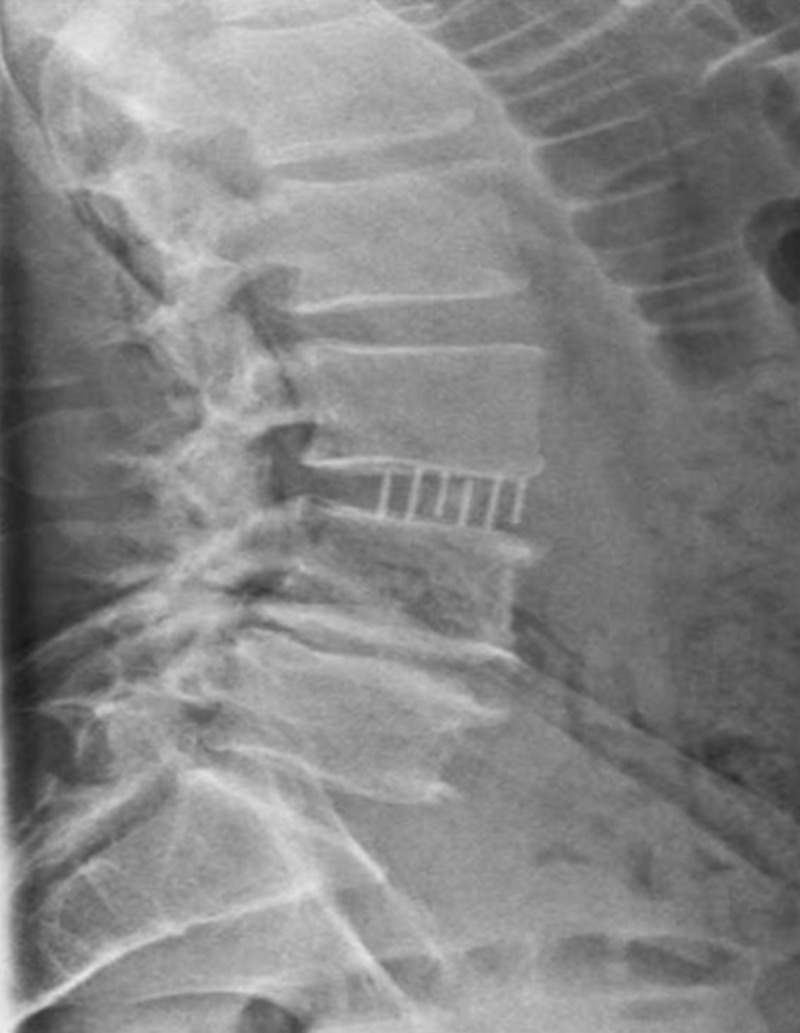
Six-month post-operative lateral standing radiograph demonstrating lateral interbody cage placement.
